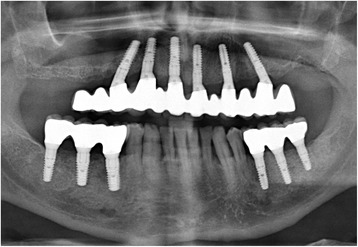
One-year post-surgery panoramic radiograph with final restoration
